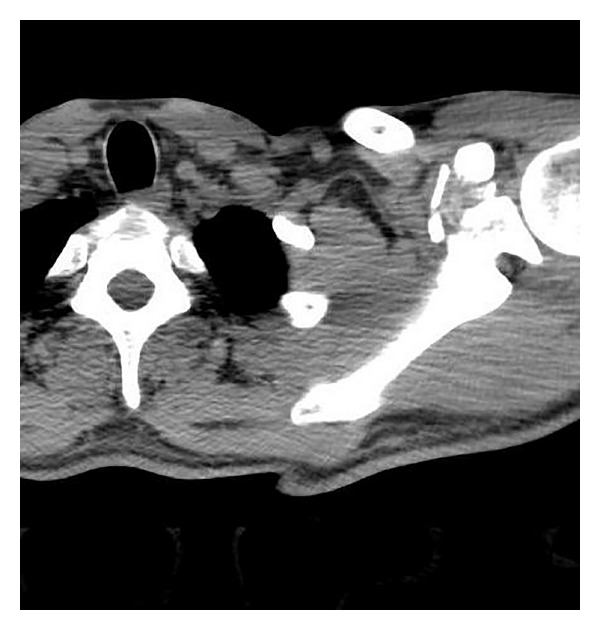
Preoperative computerised tomography (CT) image.
